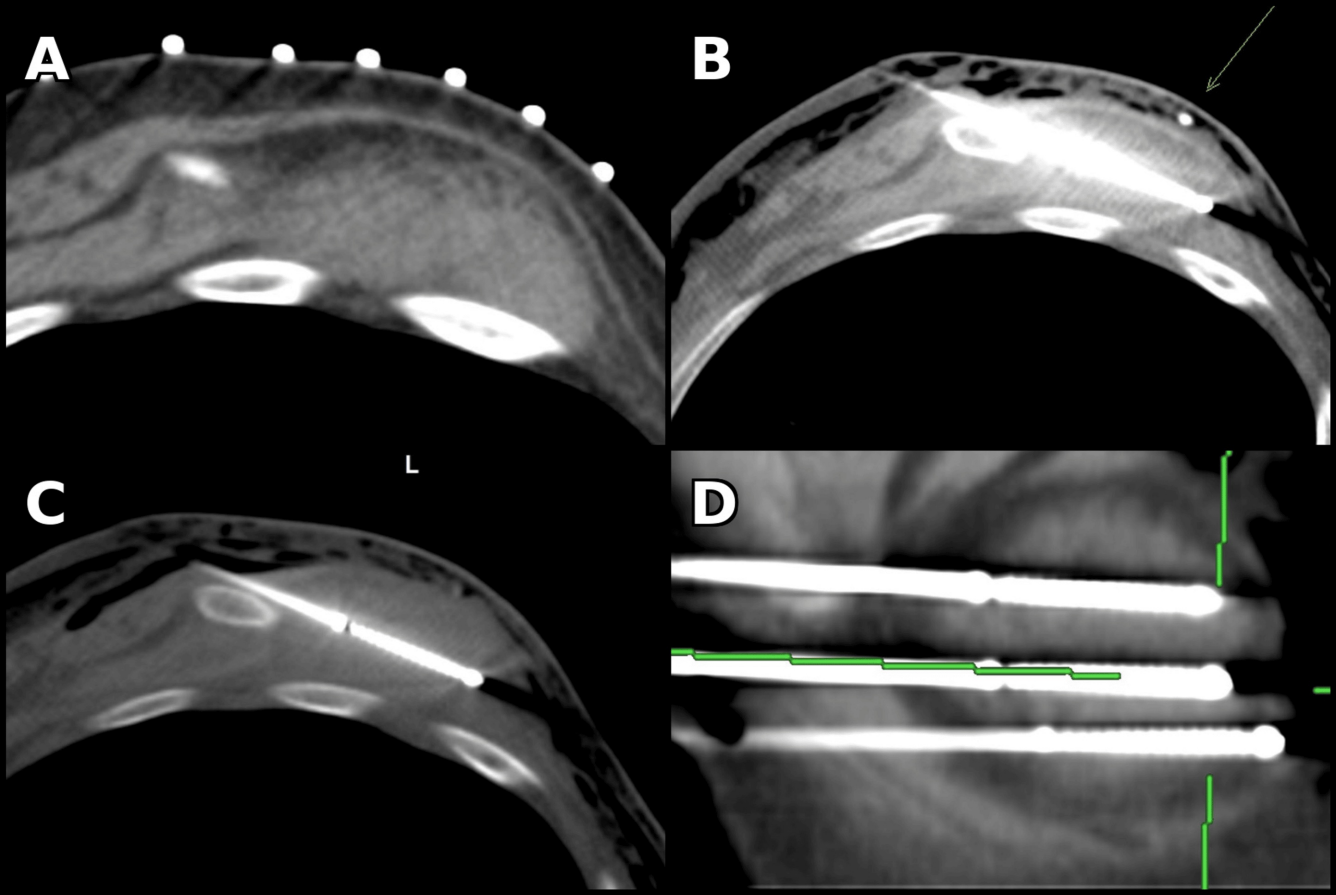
CT-guided percutaneous cryoablation of elastofibroma dorsi (A) Axial CT with grid marker used for probe trajectory planning. (B) Axial CT showing 5 Fr Yueh needle (arrow) used for pneumodissection to protect adjacent soft tissues. (C) Axial CT demonstrating probe placement within the lesion with early ice ball formation. (D) Sagittal reformatted CT image showing all three cryoprobes positioned across the mass.
